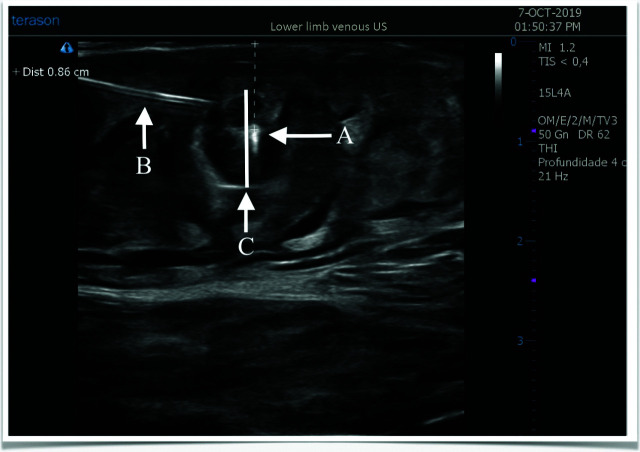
Ultrasonographic images acquired during tumescent anesthesia. Observe in the image: (A) the endolaser fiber; (B) the tumescent anesthesia needle; and (C) the tumescence halo, confirming the distance from the anterior vein wall to the skin.
